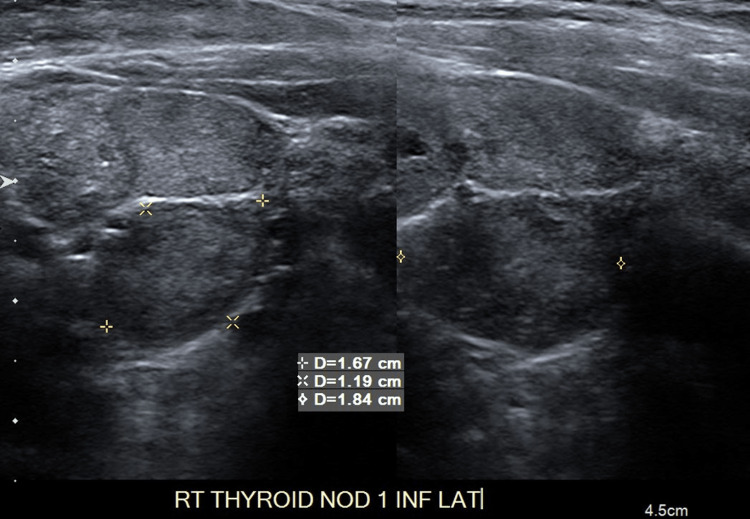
Multinodular Right (Inferolateral) Thyroid Ultrasound Lesions are marked (x) on image.
